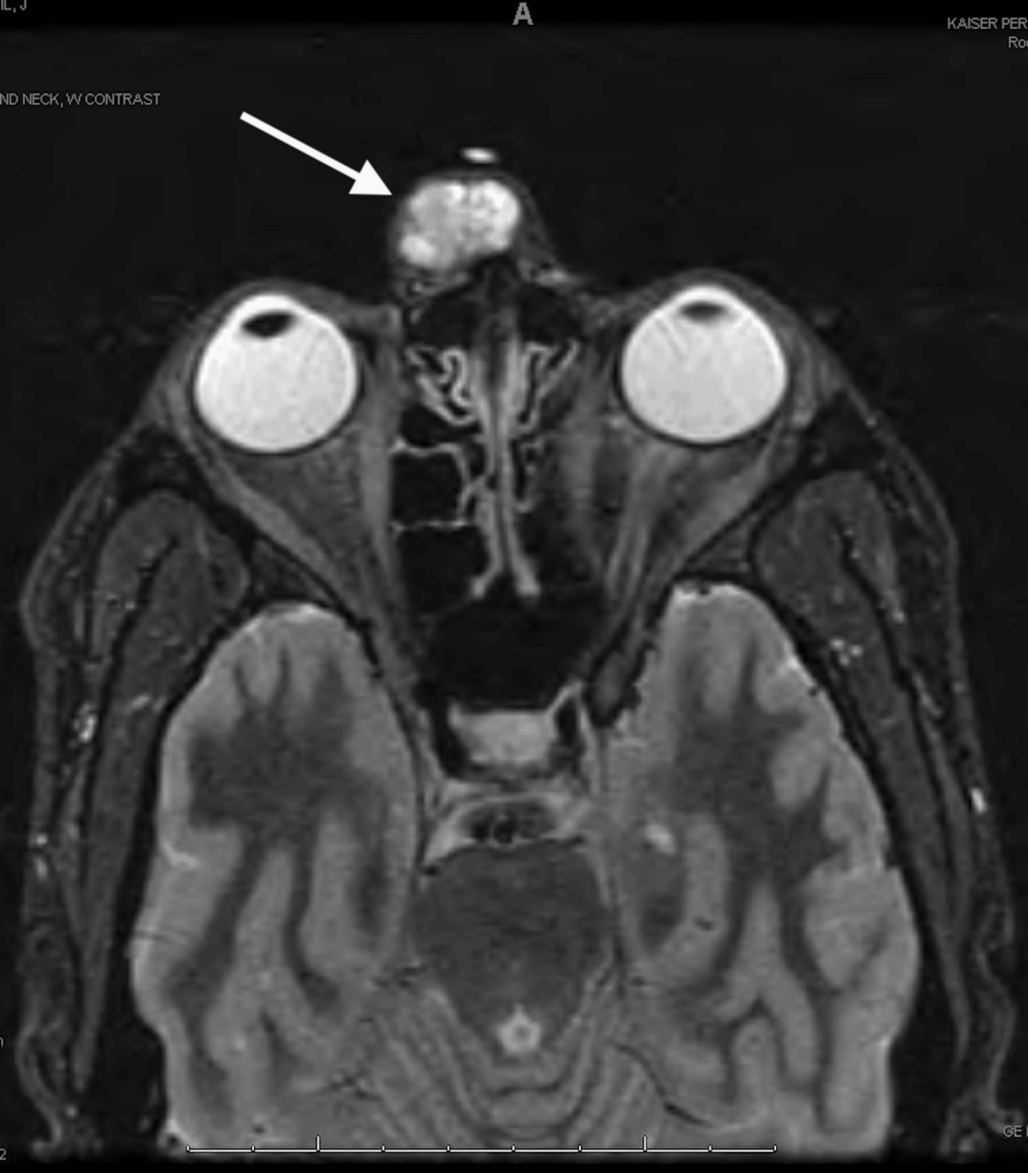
Preoperative MRI (post-contrast T2-weighted) Preoperative MRI: axial cut of a post-contrast T2-weighted image with an arrow demonstrating a contrast-enhancing mass within the glabellar region MRI: Magnetic resonance imaging
